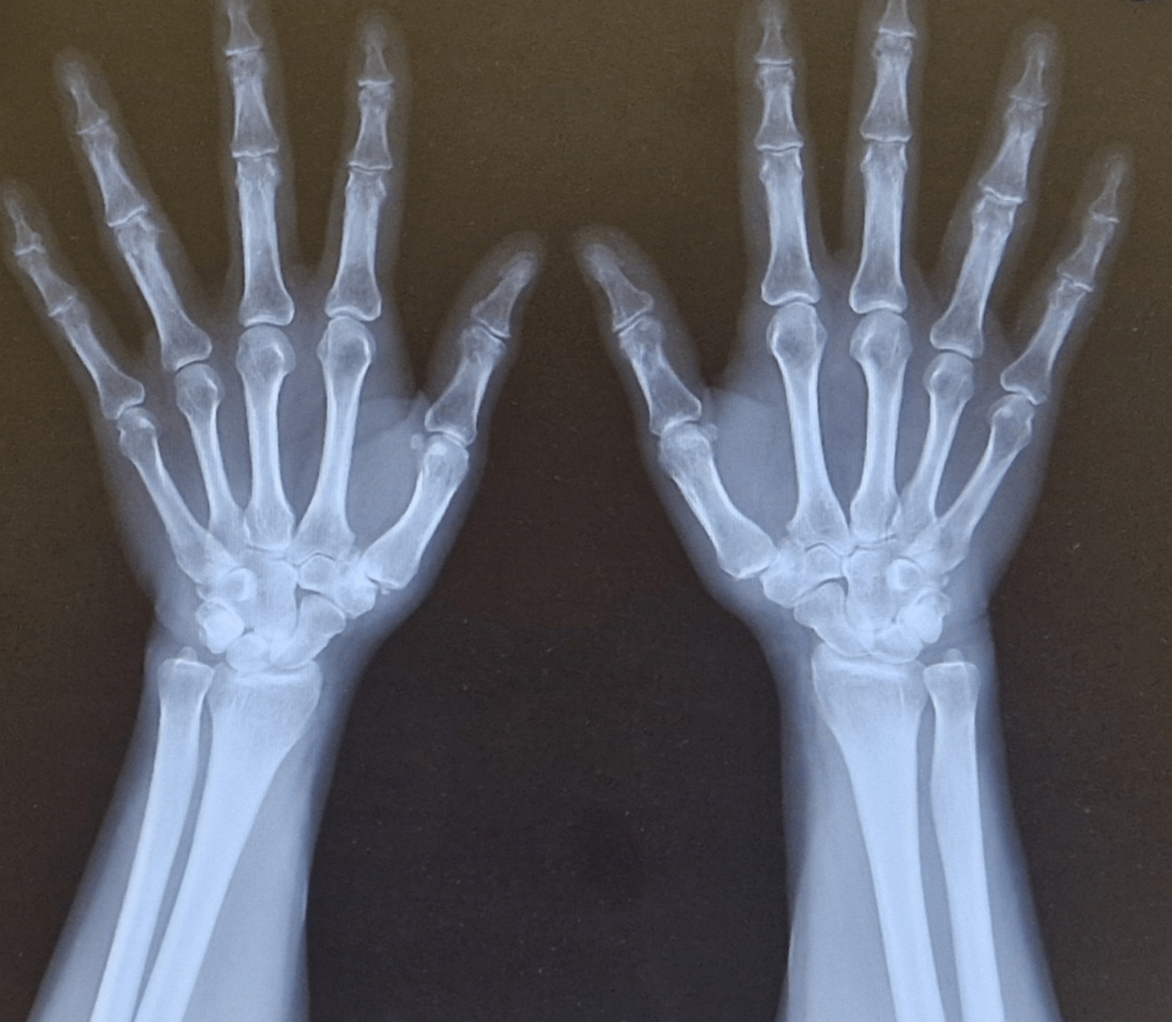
Preoperative X-ray in anteroposterior (AP) projection
